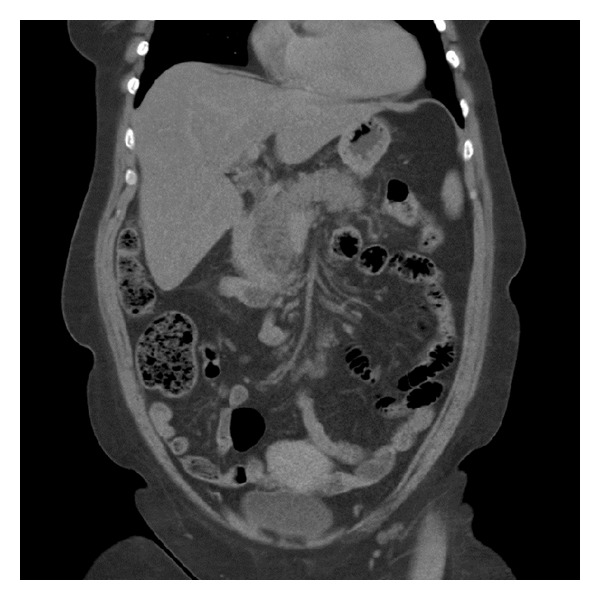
A computed tomography scan at the day of admission showing pancreatitis changes.
